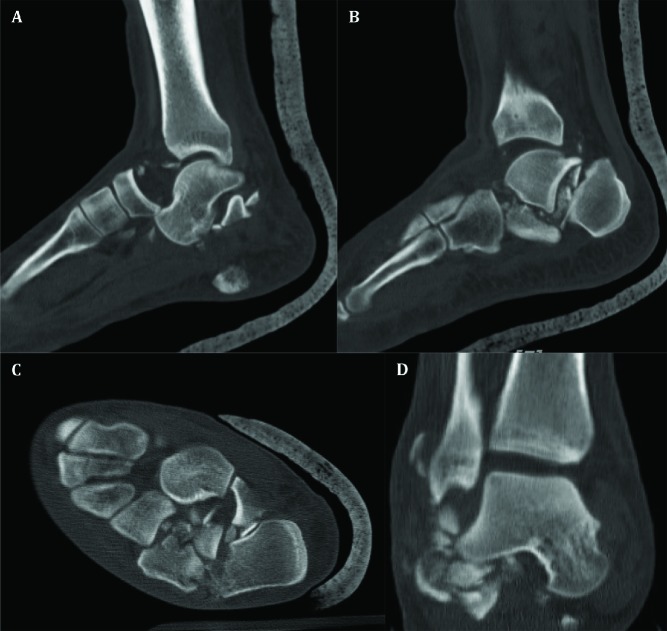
CT-scan of right ankle in sagittal (A, B), axial (C) and coronal (D) views demonstrating highly-comminuted fracture of the calcaneus, dislocated talonavicular joint, subluxation of the tibiotalar joint with multiple free fragments
